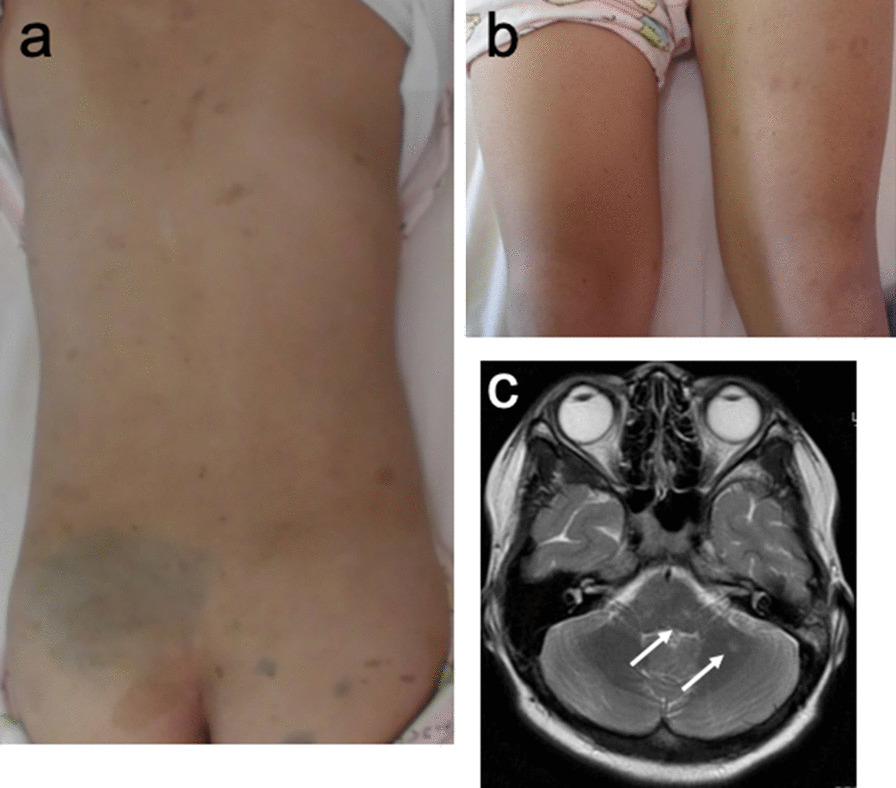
Café-au-lait macules (CALM) with irregular boundaries and uneven pigmentation on the dorsal area (a) and lower limbs (b). c Magnetic resonance imaging (MRI); arrows indicate unidentified bright objects (UBO) in the left cerebellar hemisphere
